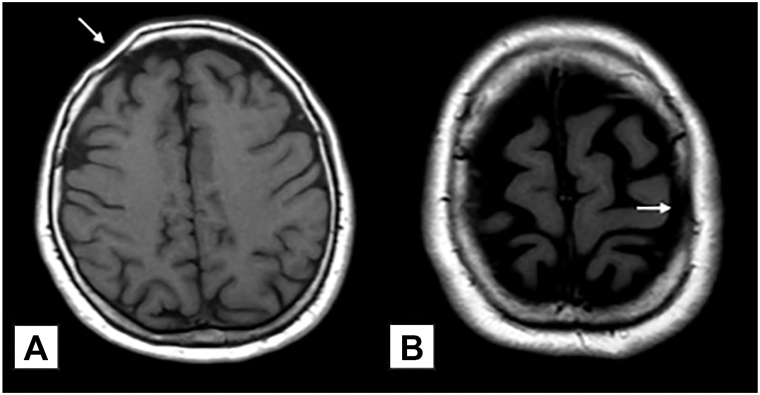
Axial brain MRI revealing severe bone loss. Subcutaneous fat is intact, favoring the diagnosis of idiopathic calvarial thinning. A, Right frontal bone thinning of the calvarium (*white arrow*). B, Left parietal bone thinning of the calvarium (*white arrow*). *MRI*, Magnetic resonance imaging.
